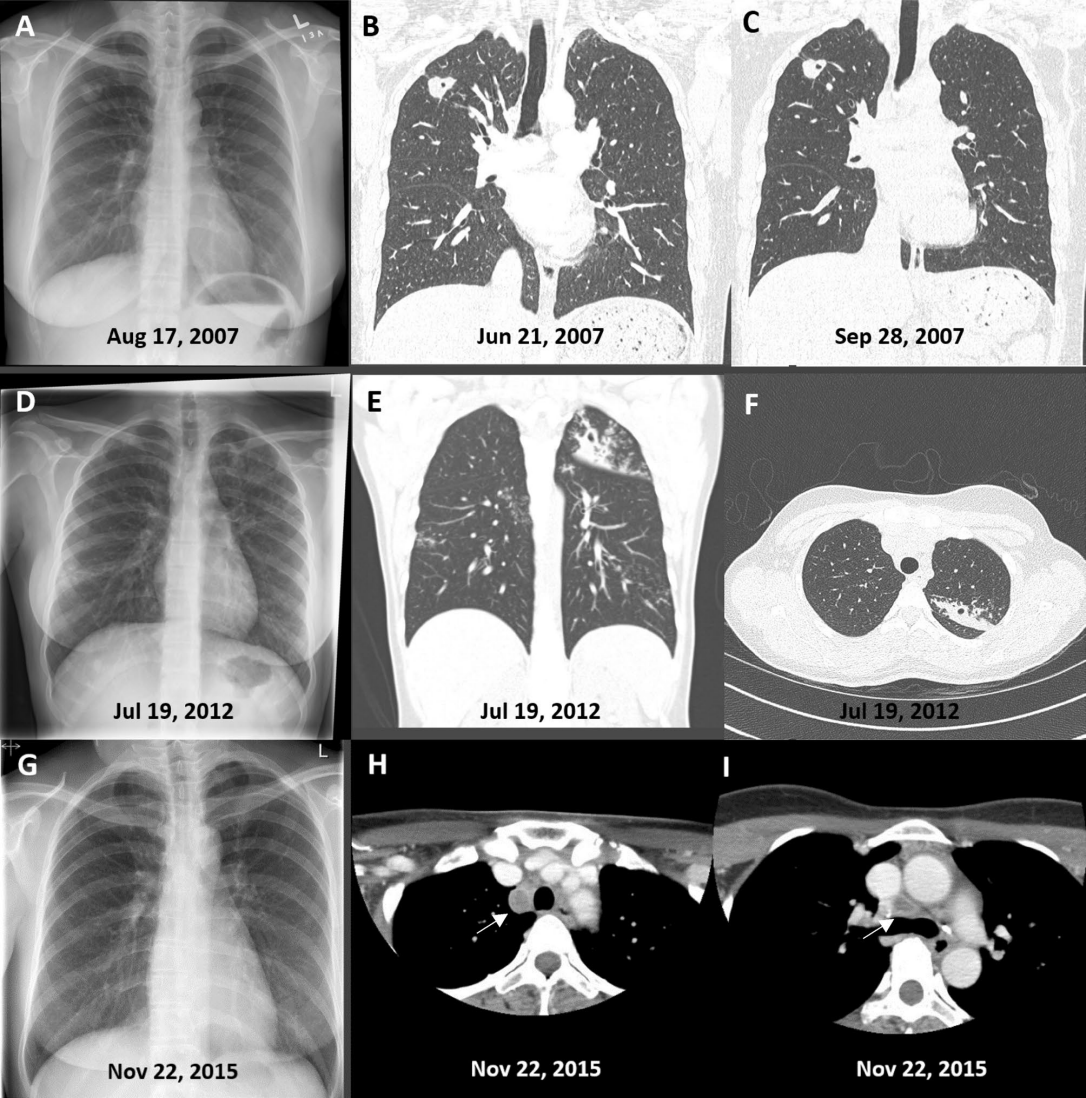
(A,B,C) Frontal chest radiograph (A) and thin Sect. (1.0 mm) coronal reformat computed tomographic (CT) images (B and C) in a patient diagnosed with smear-negative, culture-positive subclinical pulmonary tuberculosis on August 28, 2007. A small cavity is visible on CT scan but not on chest radiograph; the abnormality in the right upper lobe is stable over several months. (D,E,F) Frontal chest radiograph (D), a multiplanar reformation (MPR) coronal CT image (3 mm) (E) and a 1 mm transverse CT image through the upper thorax (F) in a patient diagnosed with smear-negative, culture-positive subclinical pulmonary tuberculosis on July 26, 2012. Cavitation is visible on CT scan but not on chest radiograph; endobronchial spread is more visible on CT scan than on chest radiograph. (G,H,I) Frontal chest radiograph (G) and 3 mm transverse CT images at the level of the aortic arch (H) and main carina (I) in a patient diagnosed with smear-positive, culture-positive subclinical pulmonary tuberculosis on November 24, 2015. An enlarged, centrally necrotic, lymph node is visible in the right paratracheal area (H) and a borderline enlarged centrally necrotic lymph node is visible just anterior to the main carina (I) on CT scan; neither was clearly visible or reported on chest radiograph.
